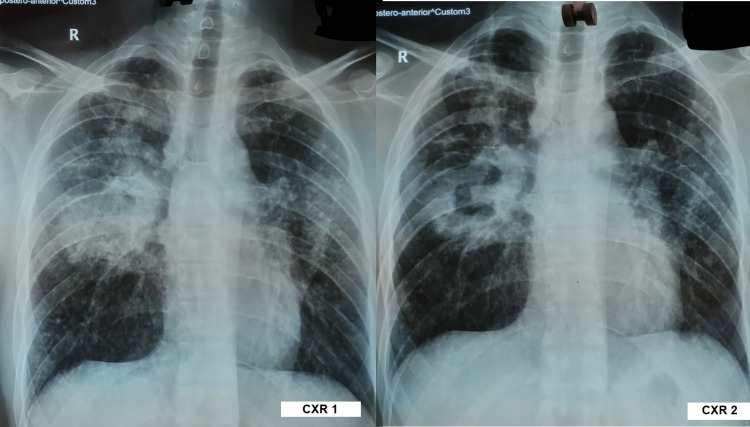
Chest x-ray images of the patient. CXR 1: chest x-ray showing ill-defined radio-opacity in right middle zone on presentation. CXR 2: chest x-ray showing an ill-defined patch of radio-opacity with area of central lucency with few patent air bronchograms after six days.
